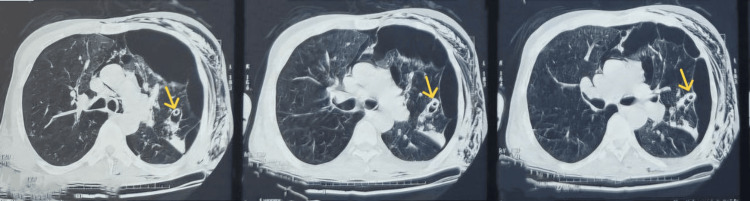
Computed tomography performed a day after chest tube insertion The scan shows the chest tube positioned in the left upper lobe (indicated by yellow arrows), with lung collapse appearing more severe than prior to the insertion
